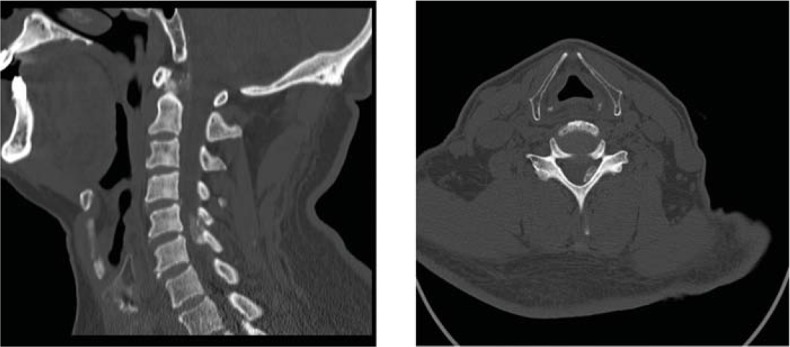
Preoperative images of CT showing spinal stenosis at the level of C5/6, mostly on left (due to the poor quality images obtained, the cervical stenosis was thought to be mostly ossification of ligamentum flavum, with no suspicion of intraspinal gout).
